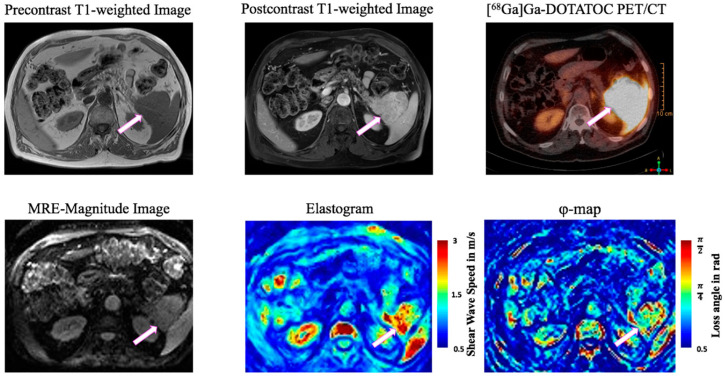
79-year-old man with pancreatic neuroendocrine tumor (PNET, white block arrow) of the pancreatic tail. Unenhanced T1-weighted image with fat saturation, postcontrast T1w image, [^68^Ga]Ga-DOTATOC positron emission tomography (PET)-CT, MRE magnitude image, elastogram and φ-map in axial plane. The tumor is characterized by low T1 signal intensity, marked contrast enhancement, PET avidity, increased stiffness (SWS) and increased loss angle of complex shear modulus (φ). Color bars represent shear wave speed (SWS) in m/s (red = high SWS, blue = low SWS) and loss angle (φ) in rad (red = high φ, blue = low φ).
